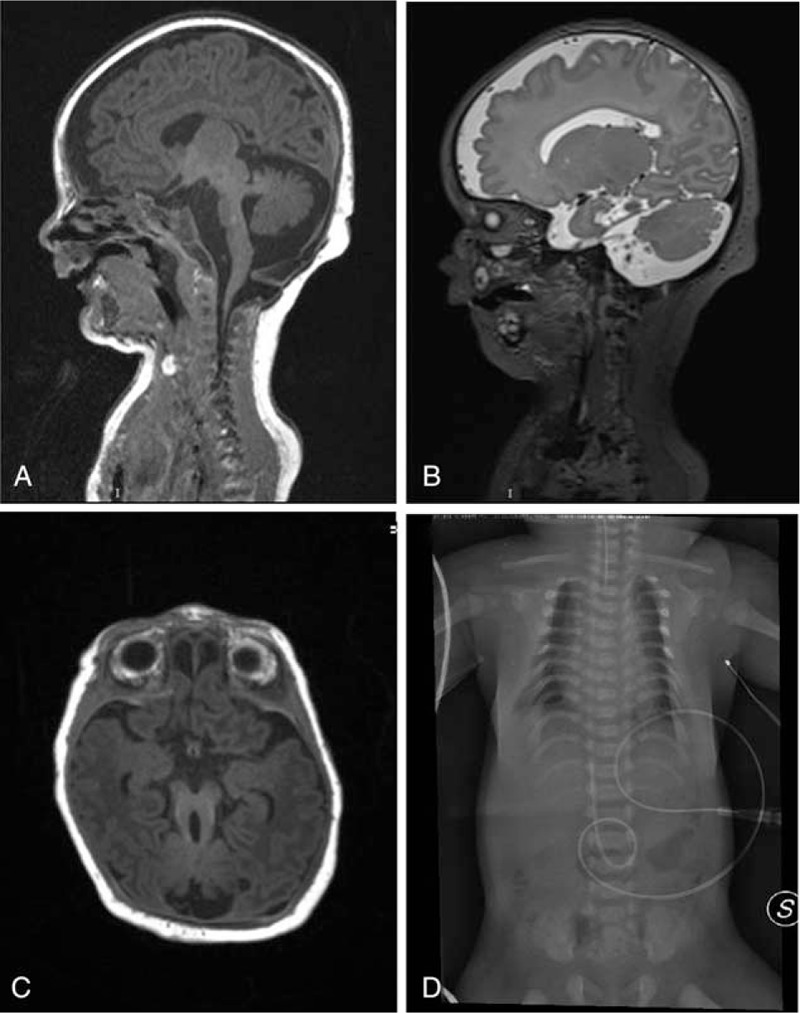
Patient 2 (A–C) Brain MRI showing cortical thickening with simplified gyral pattern, hypoplastic frontal lobes with widened fronto-insular periencephalic spaces, markedly hypoplastic cerebellum, and brain stem with elongated cerebellar peduncles and the “molar tooth” sign. (D) Skeleton X-ray showing shortened ribs, high positioned handlebar clavicles and shortened-flared upper and lower limbs metaphyses.
